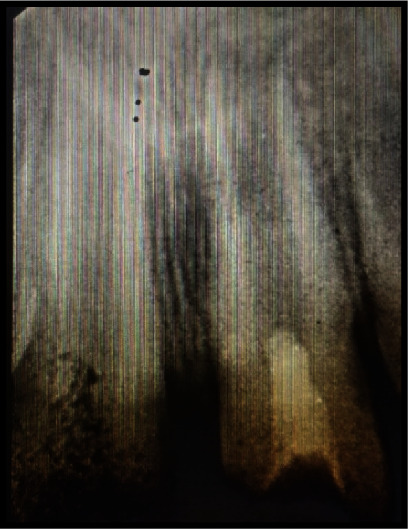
Post-operative immediate radiograph showing MTA on tooth #21.
